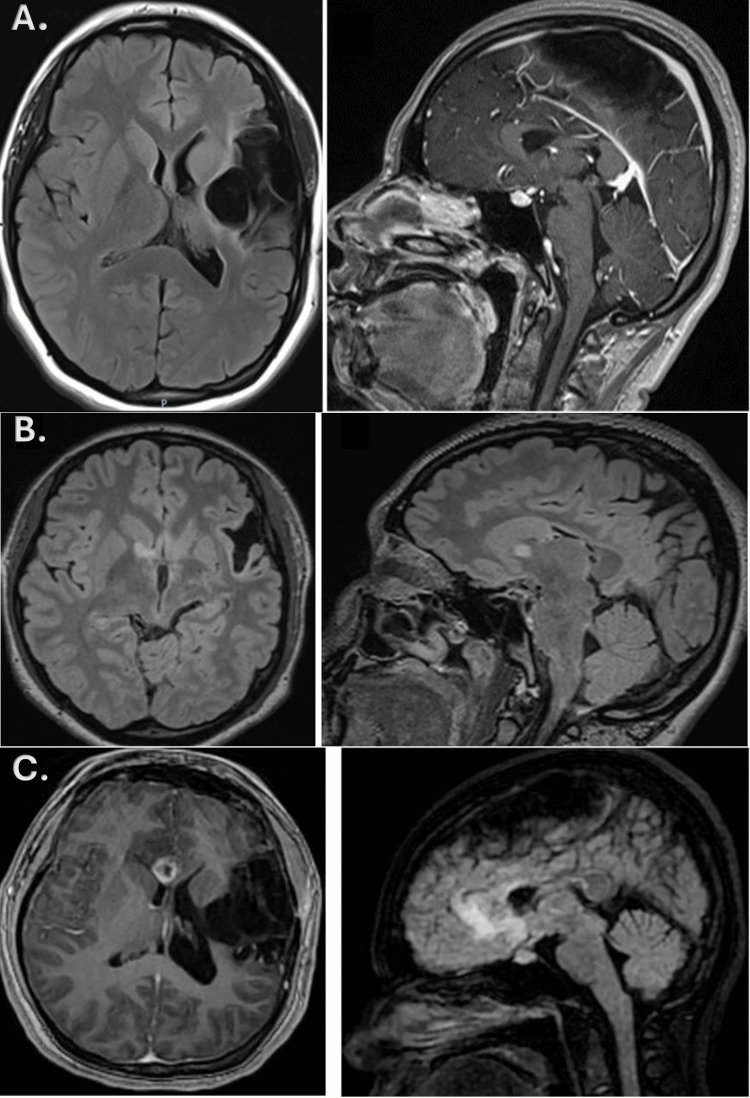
MRI findings. (A) Axial FLAIR and sagittal contrast-enhanced T1 sequences. A hypointense left frontoparietal cystic lesion can be observed, with an irregular contour and gliosis in the adjacent parenchyma. The lesion was diagnosed in childhood and caused an increase in the amplitude and retraction of the left lateral ventricle and the adjacent gyri. There was no evidence of any other structural alteration. (B) MRI findings three months after callosotomy. Axial and sagittal FLAIR sequences, with increased signal intensity at the level of the anterior arm and the knee of the right internal capsule. (C) Axial T1-contrast image showing ring-like enhancement lesions at the level of the knee of the residual corpus callosum and septum pellucidum, along with a sagittal FLAIR sequence displaying hyperintensity in the anterior cingulate cortex. FLAIR, fluid-attenuated inversion recovery; MRI, magnetic resonance imaging
